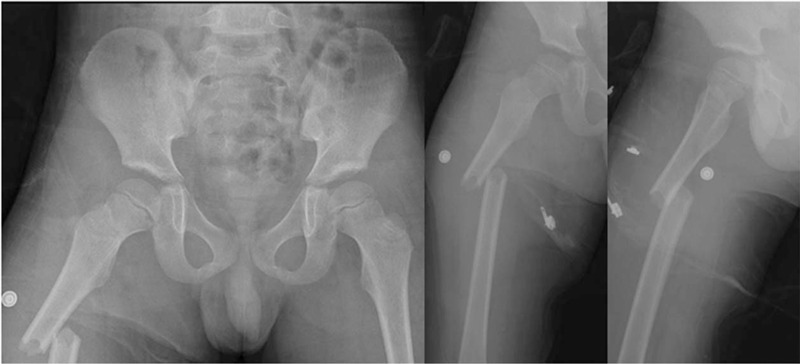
Preoperative anteroposterior and lateral x-ray showing displaced fracture of the right femoral shaft, and undisplaced femoral neck fracture.
